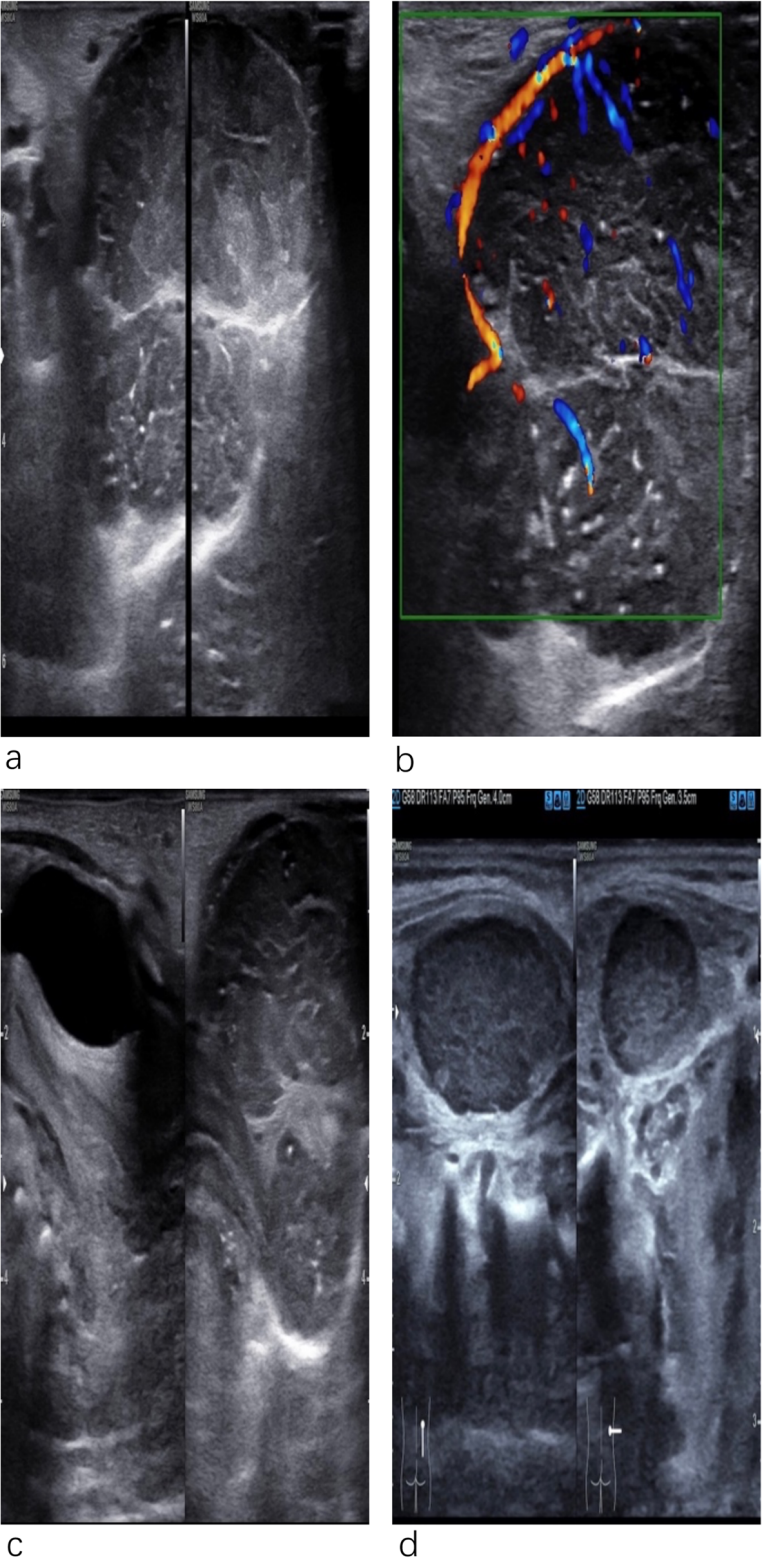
Ultrasound findings of tumor (a) Two-dimensional ultrasound showing an irregular hypoechoic solid mass in the perineum. b Color Doppler ultrasound showing thick vessels along the edge. c Two-dimensional ultrasound showing uterus and the proximal vagina. d Two-dimensional ultrasound showing a hypoechoic solid mass in the back
